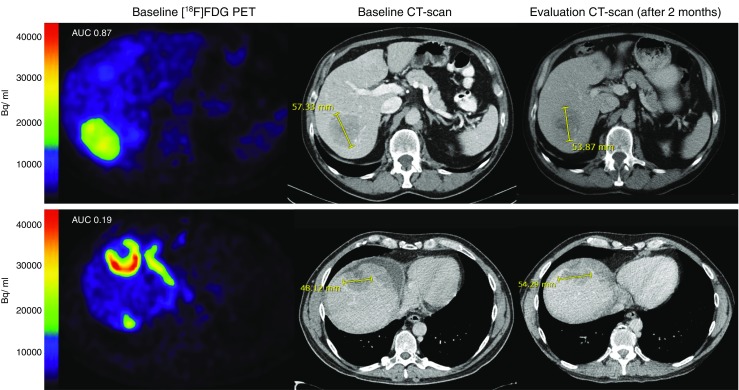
AUC-CSH and response on CT for two patents undergoing third-line cetuximab monotherapy
